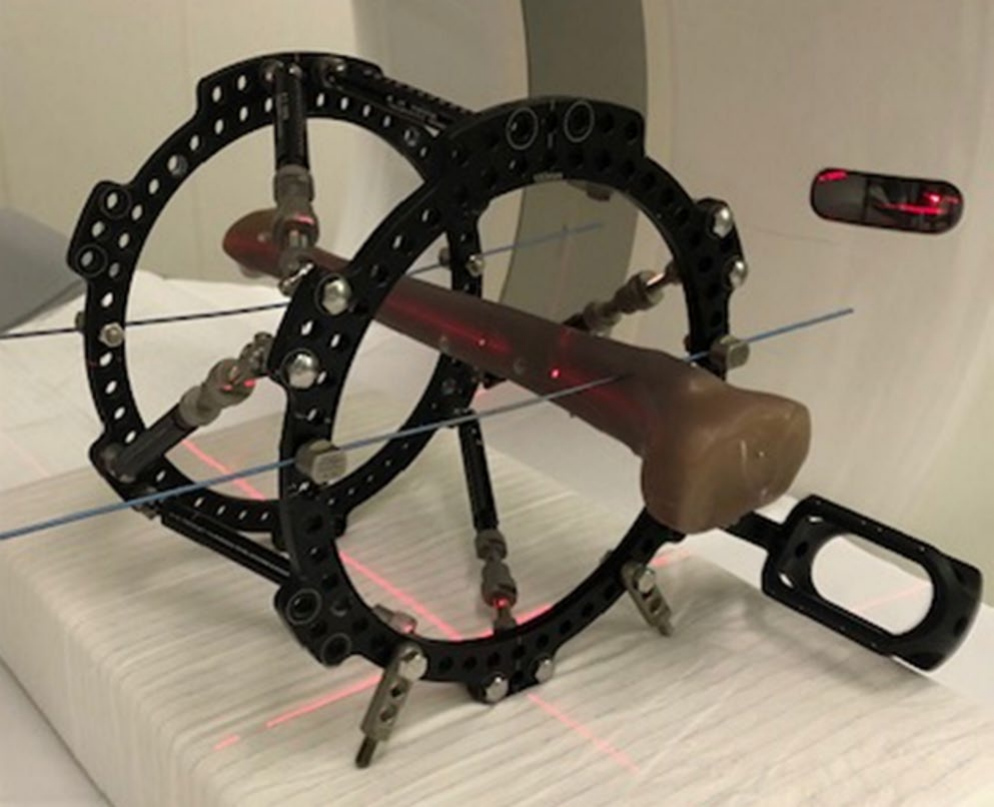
Setup for the CT (computed tomography). The tibial bone mounted in the center of the ring is positioned in the isocenter of the CT
